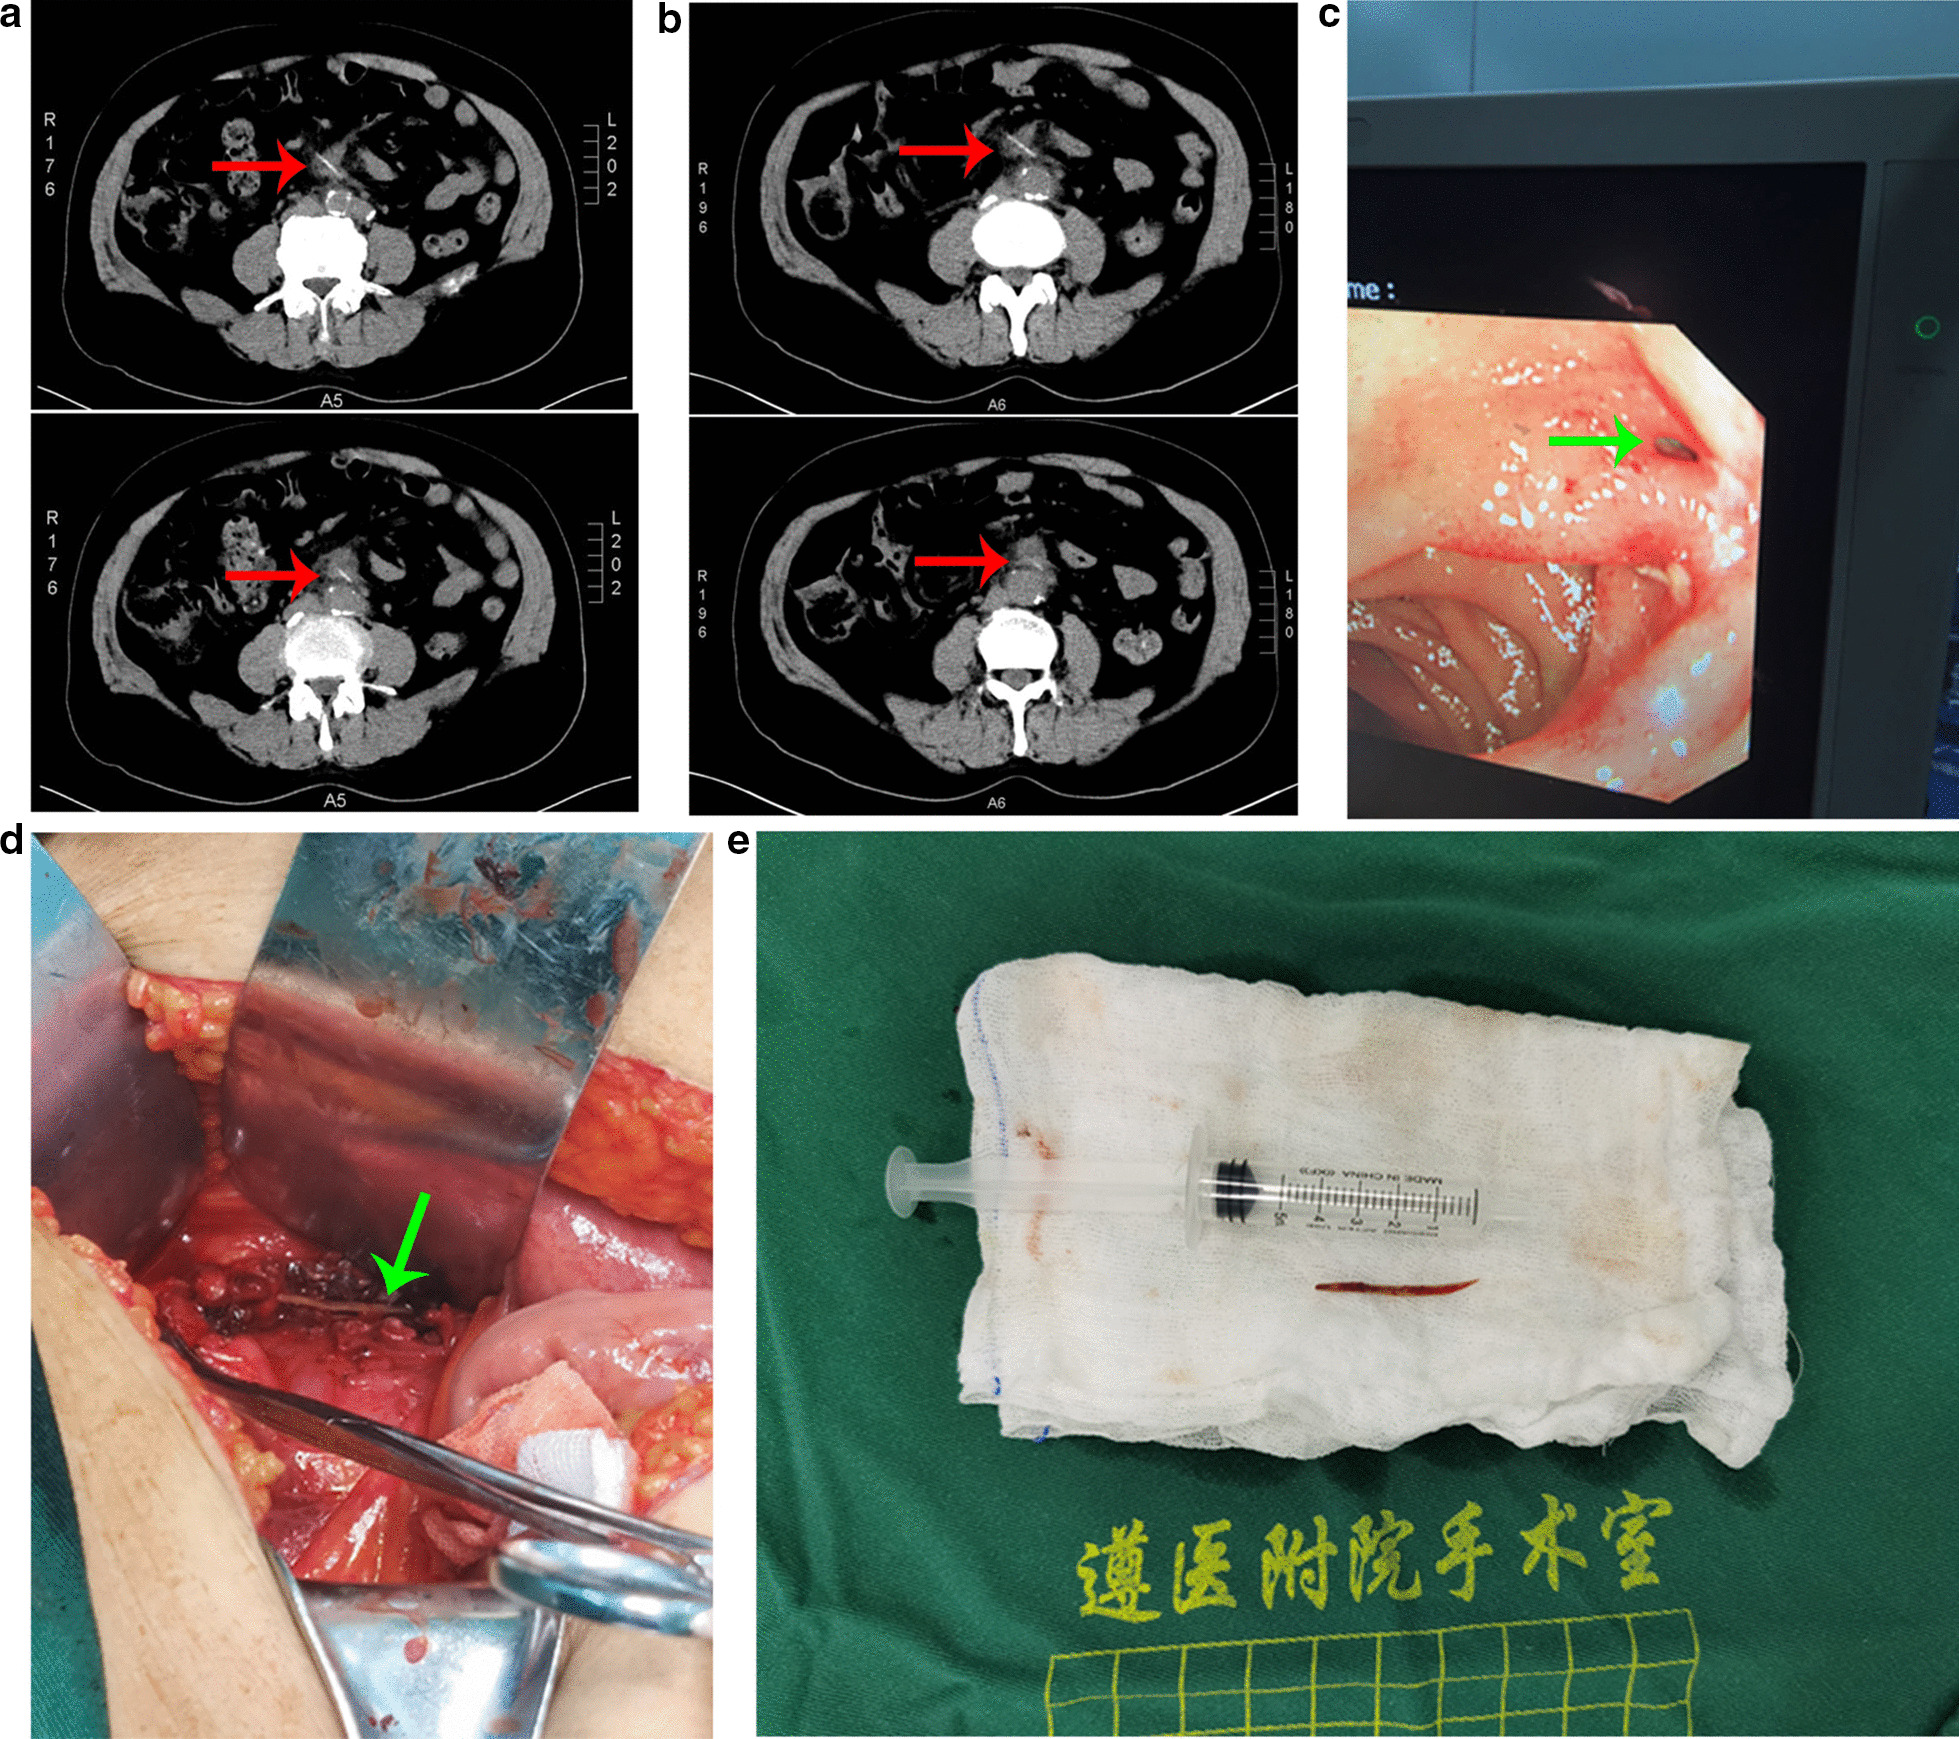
Migration of fish bones into abdominal para-aortic tissue from the duodenum after leading to duodenal perforation a Emergency abdominal computed tomography (CT) scan showed High density streaks along the horizontal anterior and lower edges of the duodenum. b Second abdominal computed tomography (CT) scan showed duodenal horizontal foreign body with peritonitis, local inclusional effusion or abscess. c Intraoperative endoscopy showed the foreign body had penetrated the intestinal wall at the junction between the horizontal and ascending parts of the duodenum. d A fishbone-like foreign body about 3 cm long was found in the inflamed tissue below the perforation. e The fishbone was like a knife
